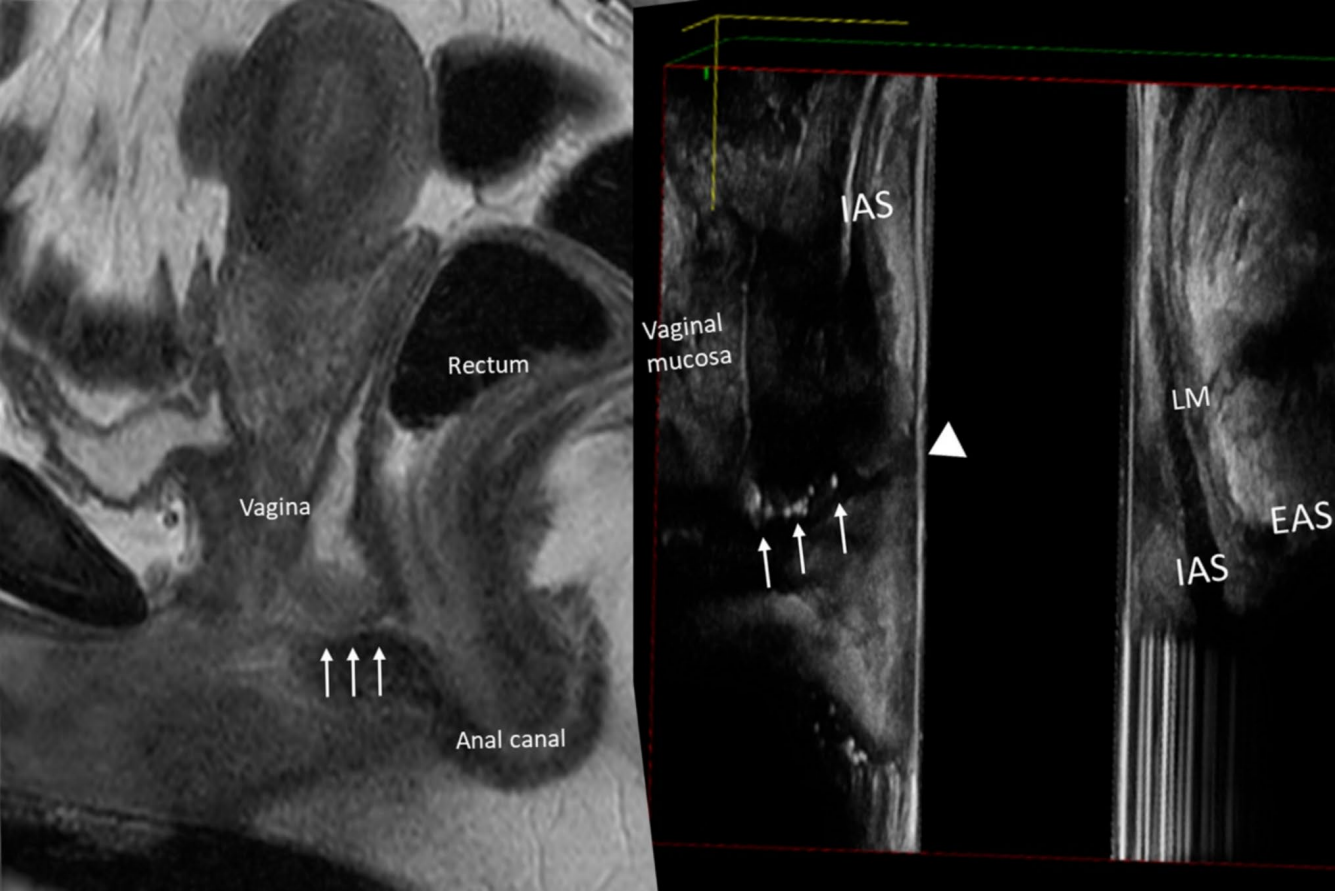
Comparison of preoperative MRI (left) and intraoperative endoanal ultrasound (right) demonstrating concordant fistula tract (arrows) between the anal canal and posterior vaginal wall. *IAS* internal anal sphincter, *EAS* external anal sphincter, *LM* longitudinal muscle
